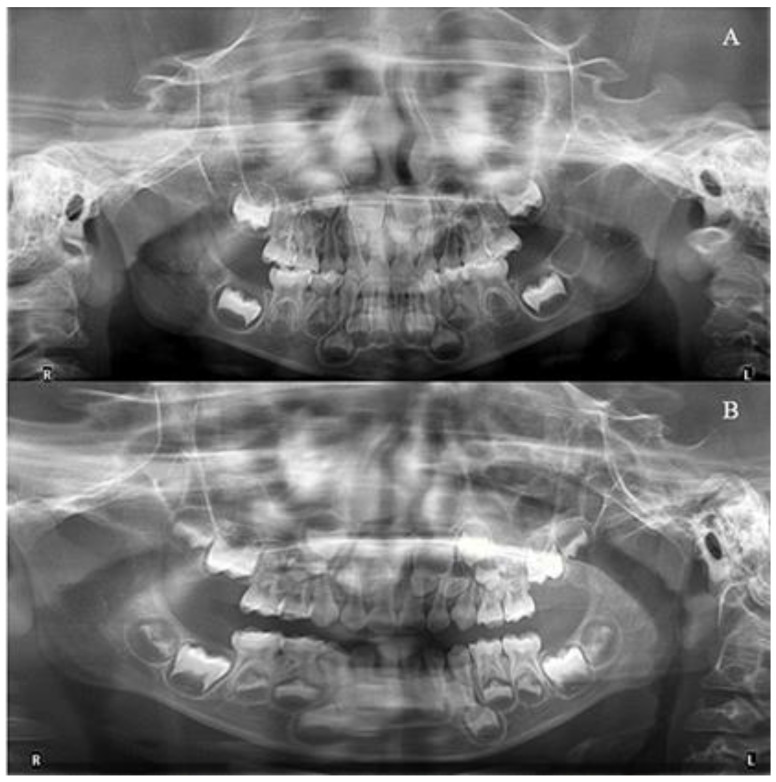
The initial and final radiographs. (A): Pre-treatment; (B): Post-treatment.
